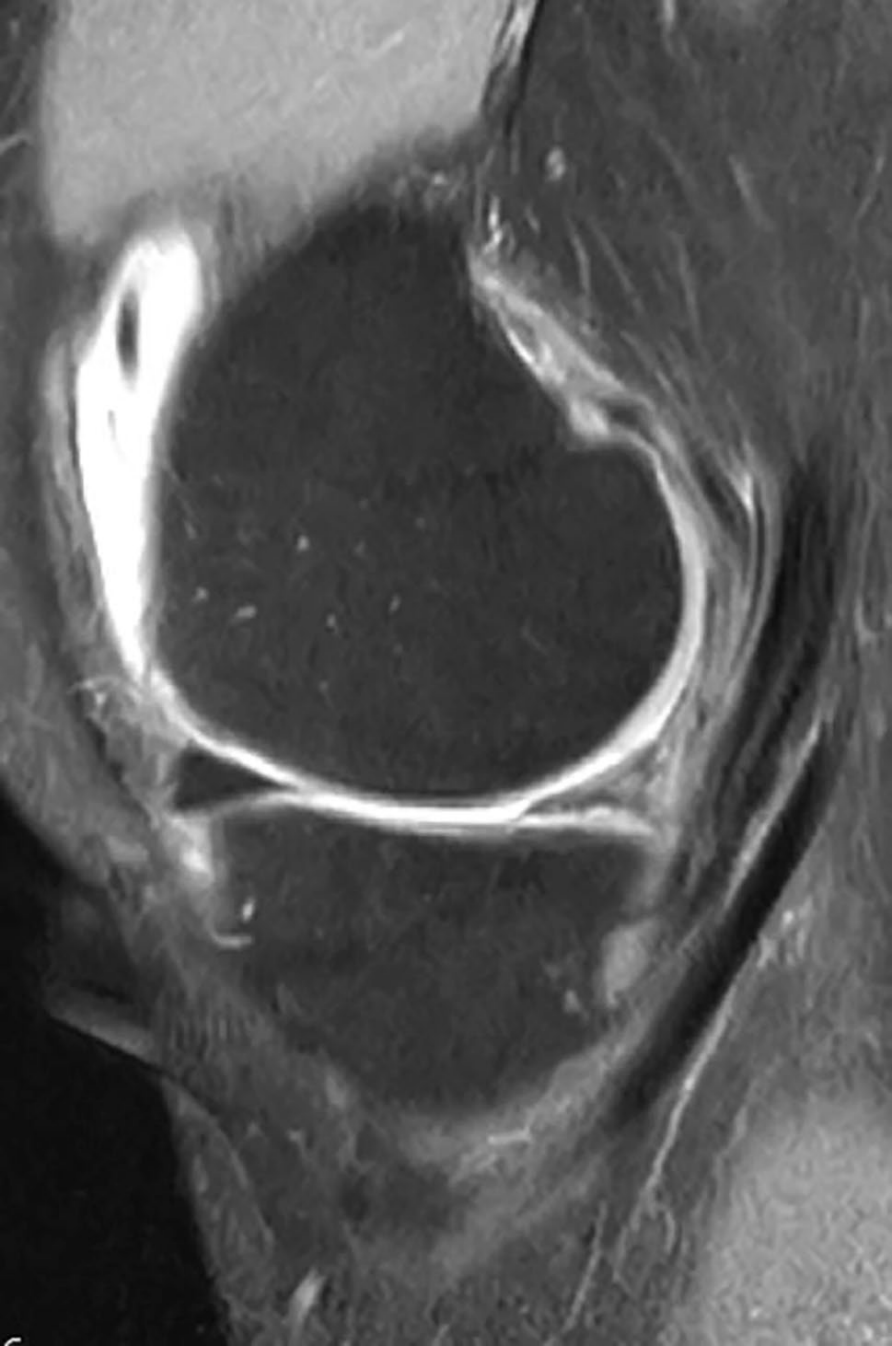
Sagittal MRI of patients 3 at 20 years of follow-up. Note that the implant is still recognizable and showing a good signal with reduced scaffold size
